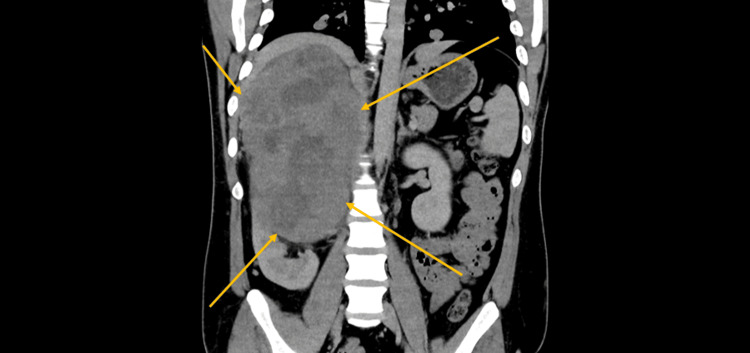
CT scan of the chest, abdomen, and pelvis revealed a 17 cm heterogeneously enhancing right-sided abdominal mass arising between the liver and right kidney, with internal necrosis, punctate calcifications, and invasion into adjacent retroperitoneal fat.
